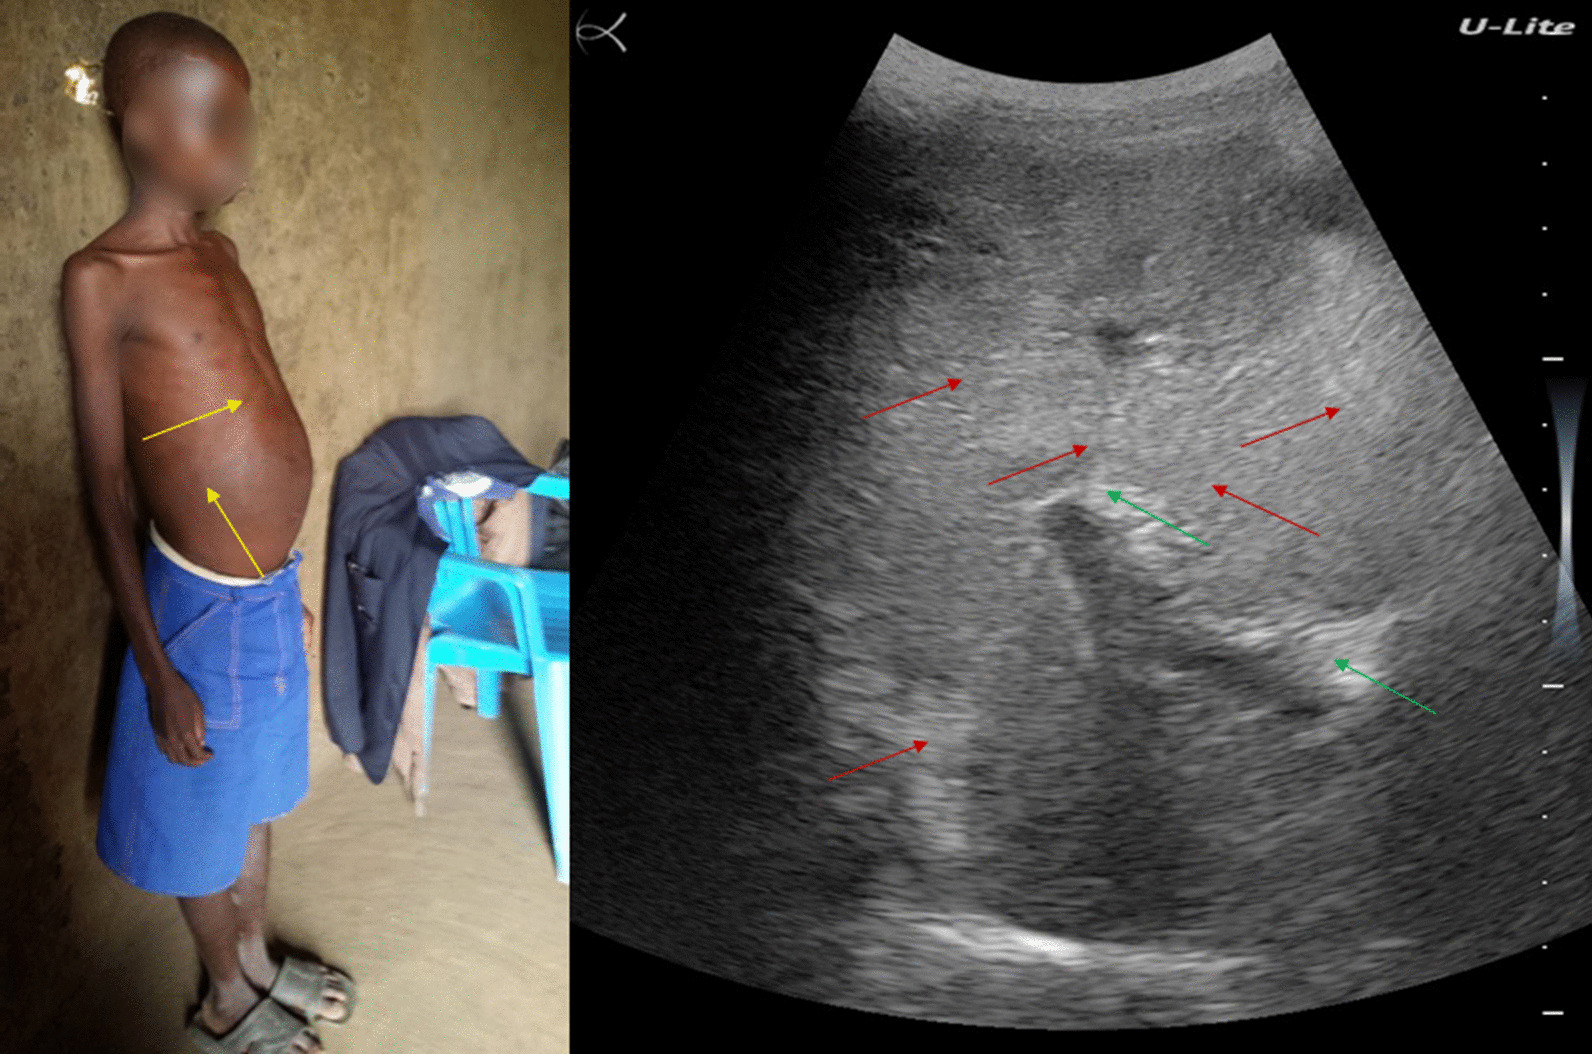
Patient #6, a cachectic young man with chronic rash spots (yellow arrows, left). Ultrasound examination (right) showing starry night sky liver pattern, liver architecture and heterogeneous echotexture of liver parenchyma (red arrow), and decreased definition of the portal vein wall (green arrow)
